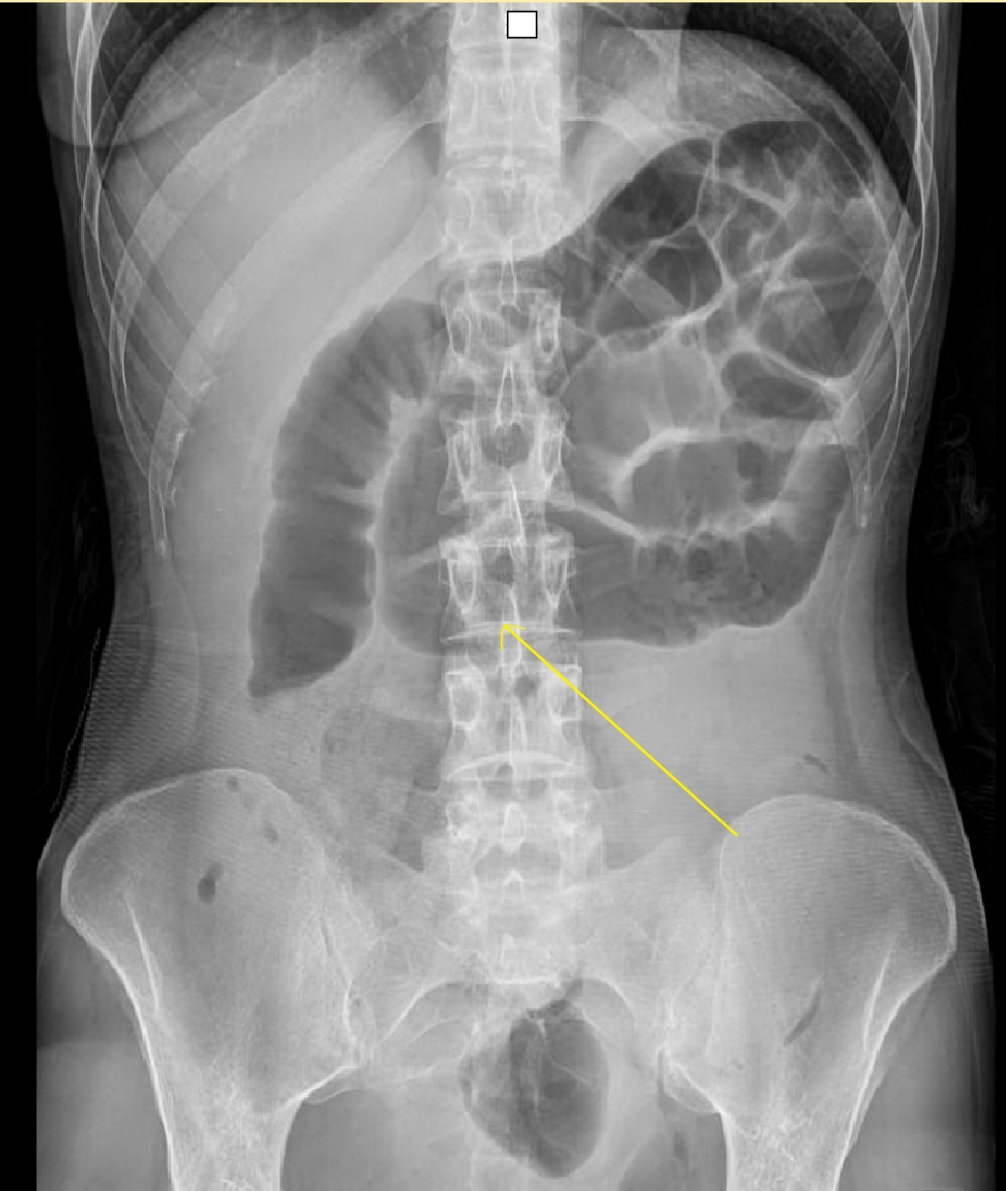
Supine abdominal X-ray A film was taken on admission for complaints of periumbilical pain and diarrhea. This was less than 18 hours before further radiological imaging. The yellow arrow shows the possible junction of the pathological couplet.
